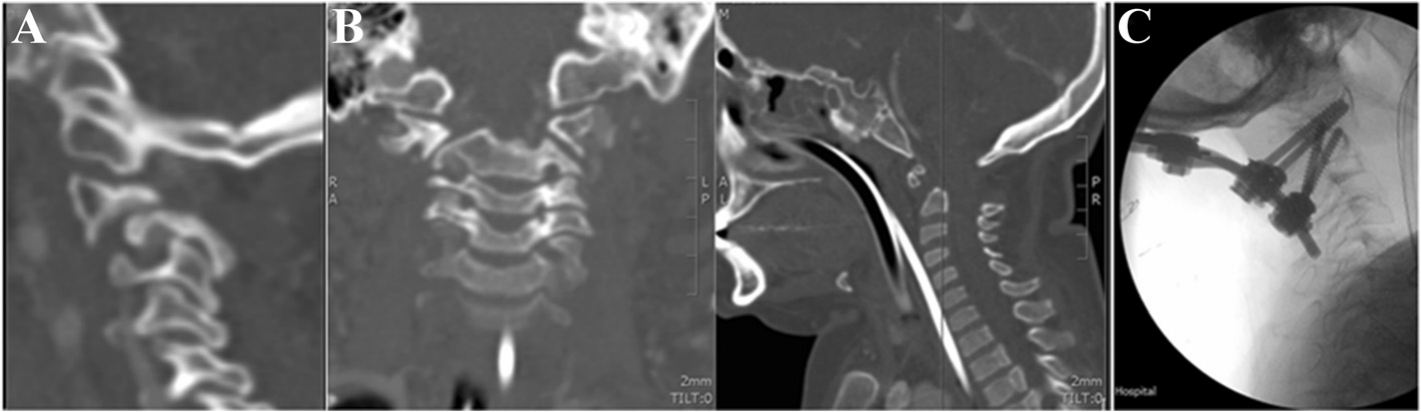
Radiological images from Case 3. a Pre-operative CT scan showing C1-2 subluxation with antero-inferior subluxation of C1 lateral mass on C2; (b) pre-operative MRI images showing coronal and sagittal views of C2 demonstrating congenital dens hypoplasia with central dip typical of embryological abnormality and absence of posterior arch of C1; (c) post-operative XR image showing occipital C2 fixation following C1-2 reduction (C1 now in line with C2)
